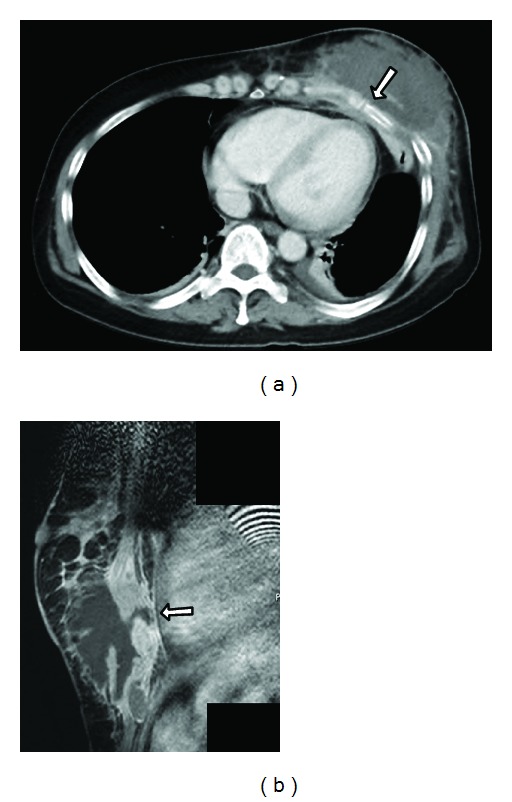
(a) Computed tomography (CT) showing a large mass in the lower region of the left breast. The fifth rib under the mass is fractured (arrow). (b) T1-weighted magnetic resonance imaging reveals contrast enhancement from the border of the mass to the pleura (arrow).
